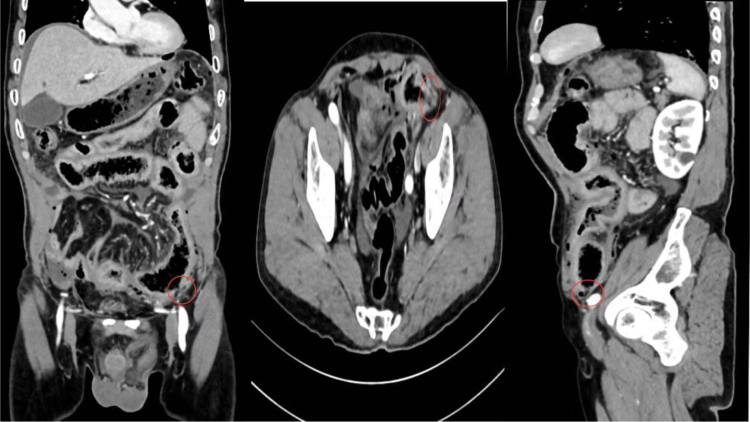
Preoperative abdominal CT showed the site of small bowel perforation. CT = computed tomography.
